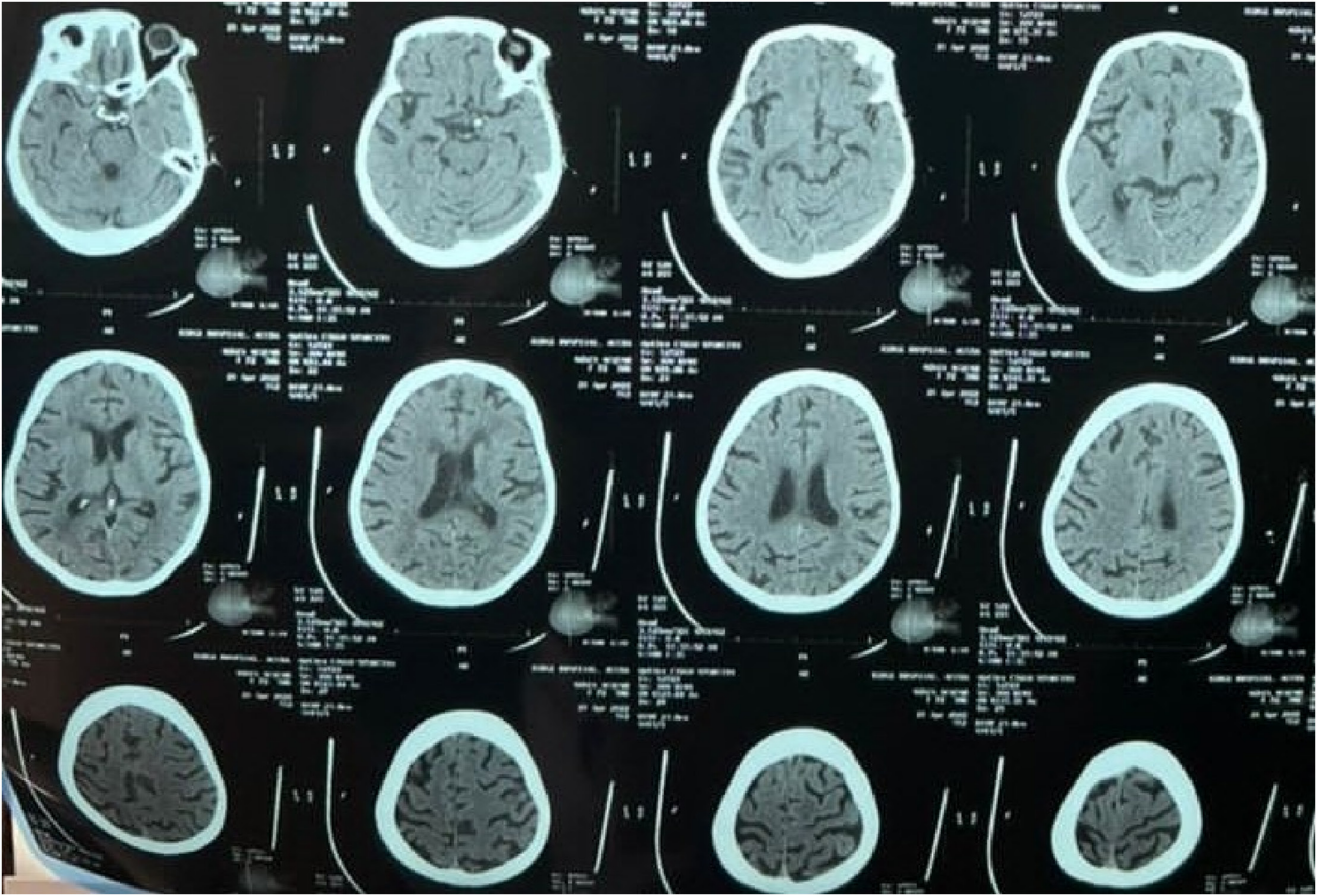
CT scan of the head: initial head CT scan on presentation and admission
